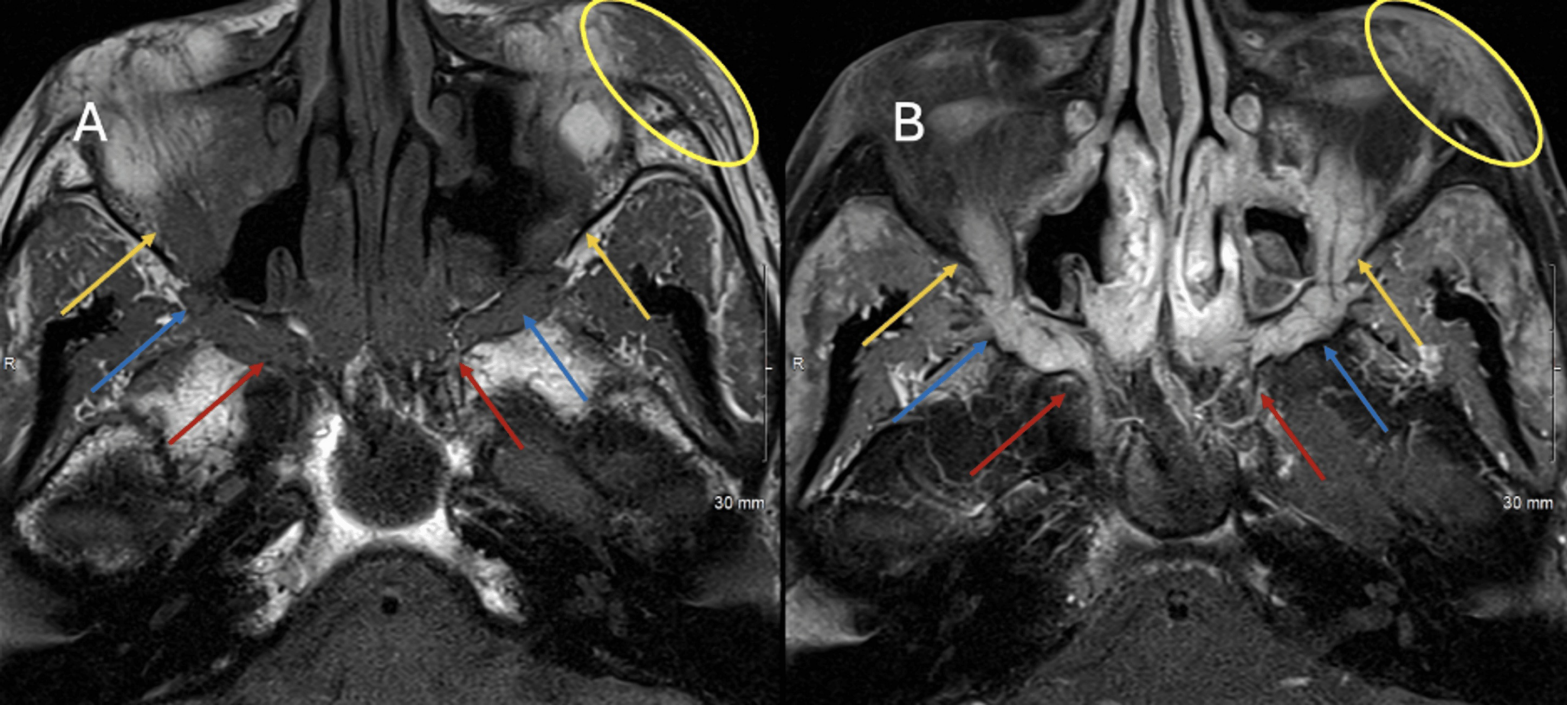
Axial MRI scans of orbits with and without contrast Axial T1 weighted MR image without contrast (A) and axial fat-saturated T1 weighted MR image at the same level with contrast (B). There is enlargement with thickening of the left lacrimal gland (yellow oval) related to IgG4-RD. Furthermore, there is thickening with an enhancement of the infraorbital nerves bilaterally within the infraorbital nerve canal (orange arrows), pterygopalatine fossa (blue arrow), and entering the foramen rotundum (red arrows). On the right side, infraorbital nerve enhancement extends through the foramen rotundum into the expected location of Meckel's cave. IgG4-RD - immunoglobulin G4-related disease
